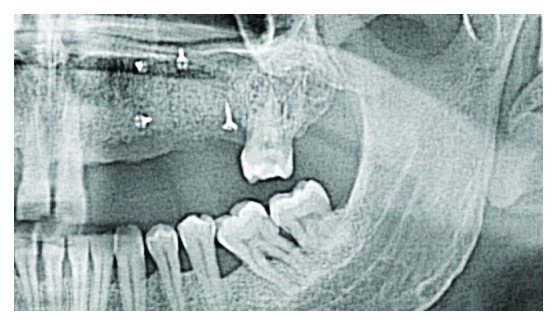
Orthopantomography after the surgery.
